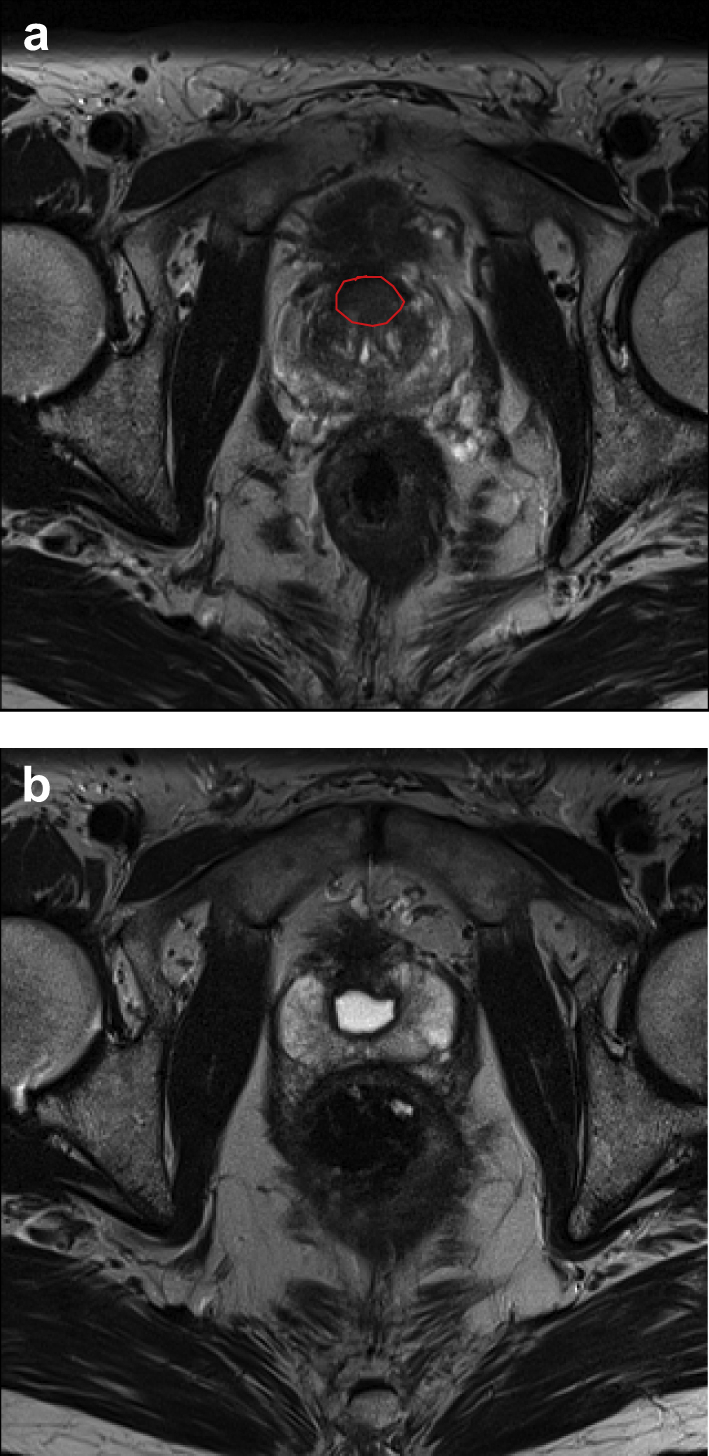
Magnetic resonance imaging (MRI) appearances before and after focal high-intensity focussed ultrasound treatment to the prostate. A T2-weighted prostate MRI image of a man with presenting prostate-specific antigen of 7.7 ng/ml is given in (a). A scanner with a 1.5 Tesla magnet and a pelvic phased array coil was used to capture images. An anterior prostate tumour is indicated by the red circle. Transperineal template prostate biopsies confirmed high volume Gleason 3 + 3 disease. The patient underwent focal high-intensity focussed ultrasound treatment of the tumour. Six months after treatment, the patient underwent repeat MRI and the T2-weighted MRI image obtained is given in (b). The ablation cavity can be seen with no evidence of residual cancer. Prostate-specific antigen at this time was 1.1 ng/ml.
